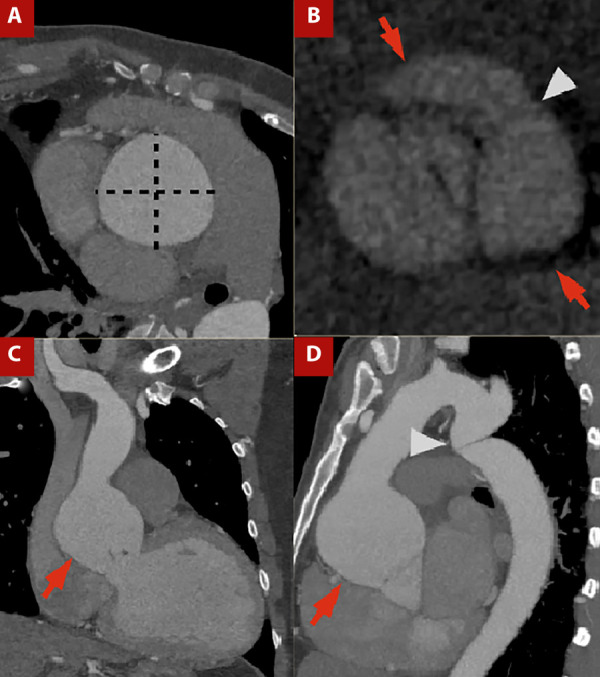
A) Contrast-enhanced CT angiography of the thoracic aorta with cardiac gating, orthogonal plane of the ascending aorta. B) Aortic valve plane in ventricular systole. C) Coronal view and D) Sagittal view.Ascending aortic dilatation is evident, with a maximum diameter of 64 × 64 mm measured in the orthogonal plane (dashed line in A), involving the sinotubular junction (arrows in C and D). A bicuspid aortic valve is present, with fusion of the right and left coronary sinuses (arrows in C) and visualization of the raphe (arrowhead in C). The patient also had thoracicaortic coarctation (arrowhead in D).
